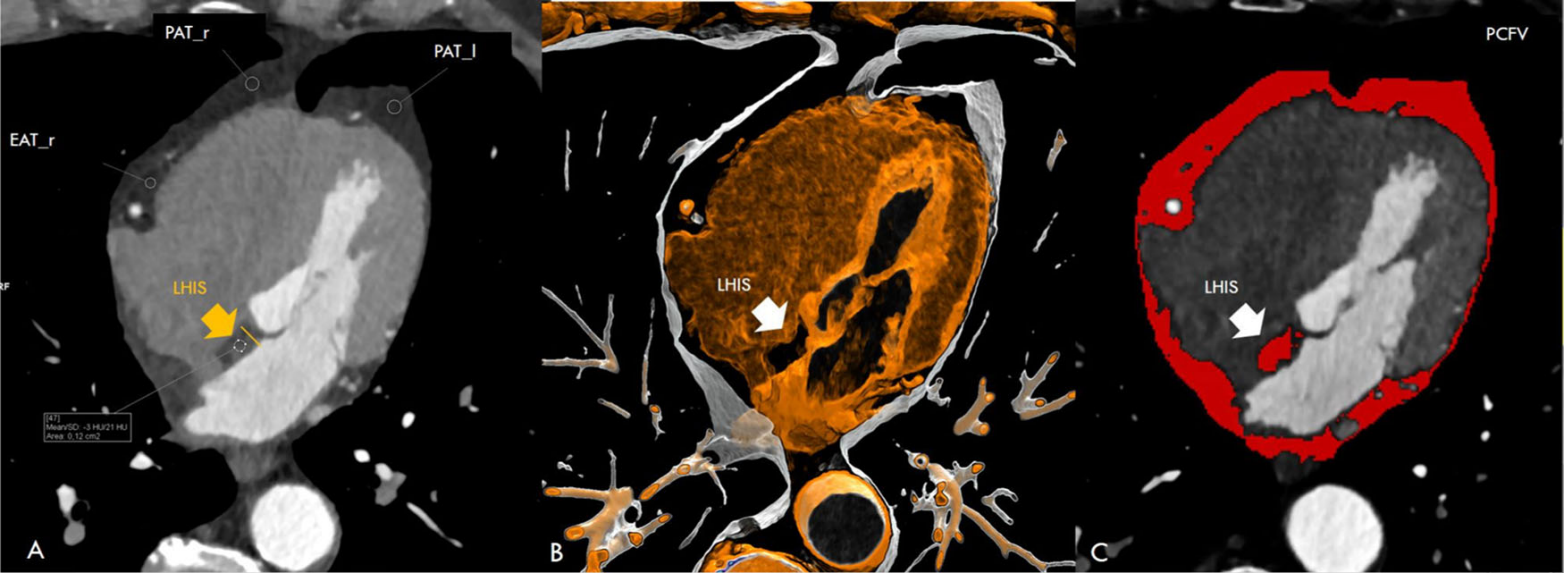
Quantification of LHIS density and size (A, B) and total PCFV by CT (C, by using SyngoVIA Frontiers, cardiovascular risk assessment) prior to editing and exclusion of LHIS in 66-years-old male (BMI 28.4 kg/cm^2^) male referred to coronary CTA. Axial CT images show LHIS (arrow), quantification of LHIS, EAT, and PAT density, and measurement of LHIS width (orange line, A)
